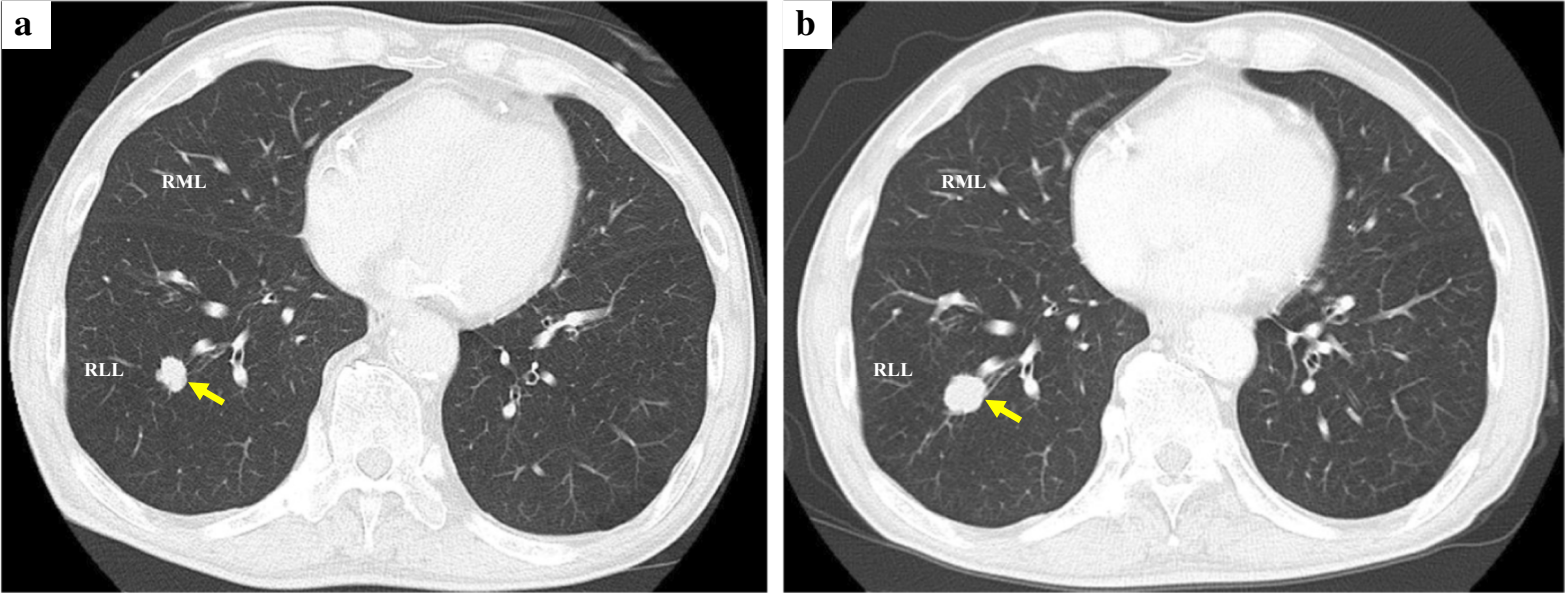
Axial section of computed tomography findings. The tumor is located in S9 of the right lung (arrow). a Four months before lung resection. b One month before lung resection. *RLL* right lower lobe; *RML* right middle lobe
